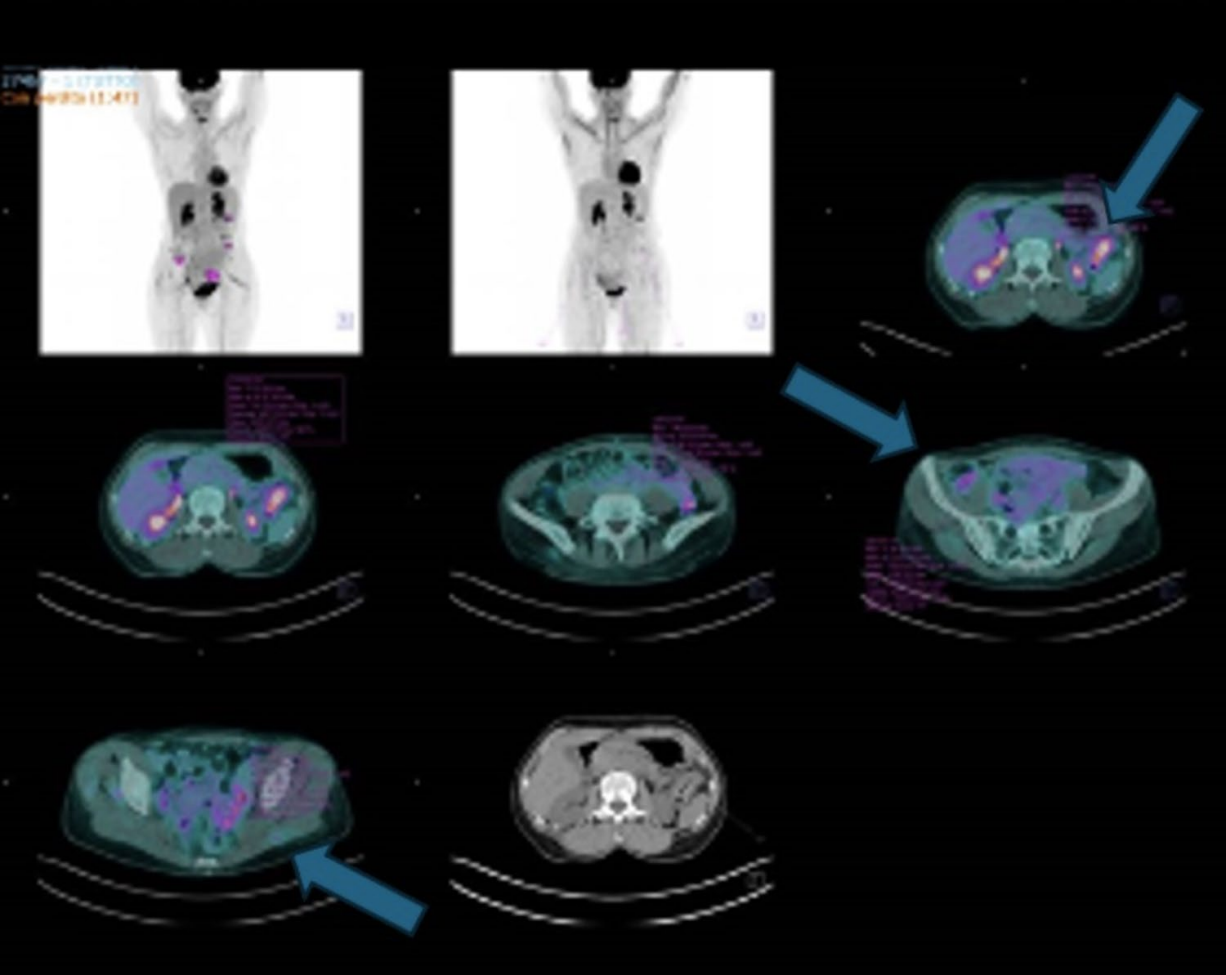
PET-CT scan performed in December 2024. The arrows show *“Increase in both the extent and intensity of radiopharmaceutical uptake of the known left colic lesion just inferior to the splenic flexure (SUV 7,73). Appearance of glucose hypermetabolism in the colon in the bilateral iliac fossa and at the level of the sigmoid colon. These findings are suspicious for localization of metabolically active secondary disease”*
